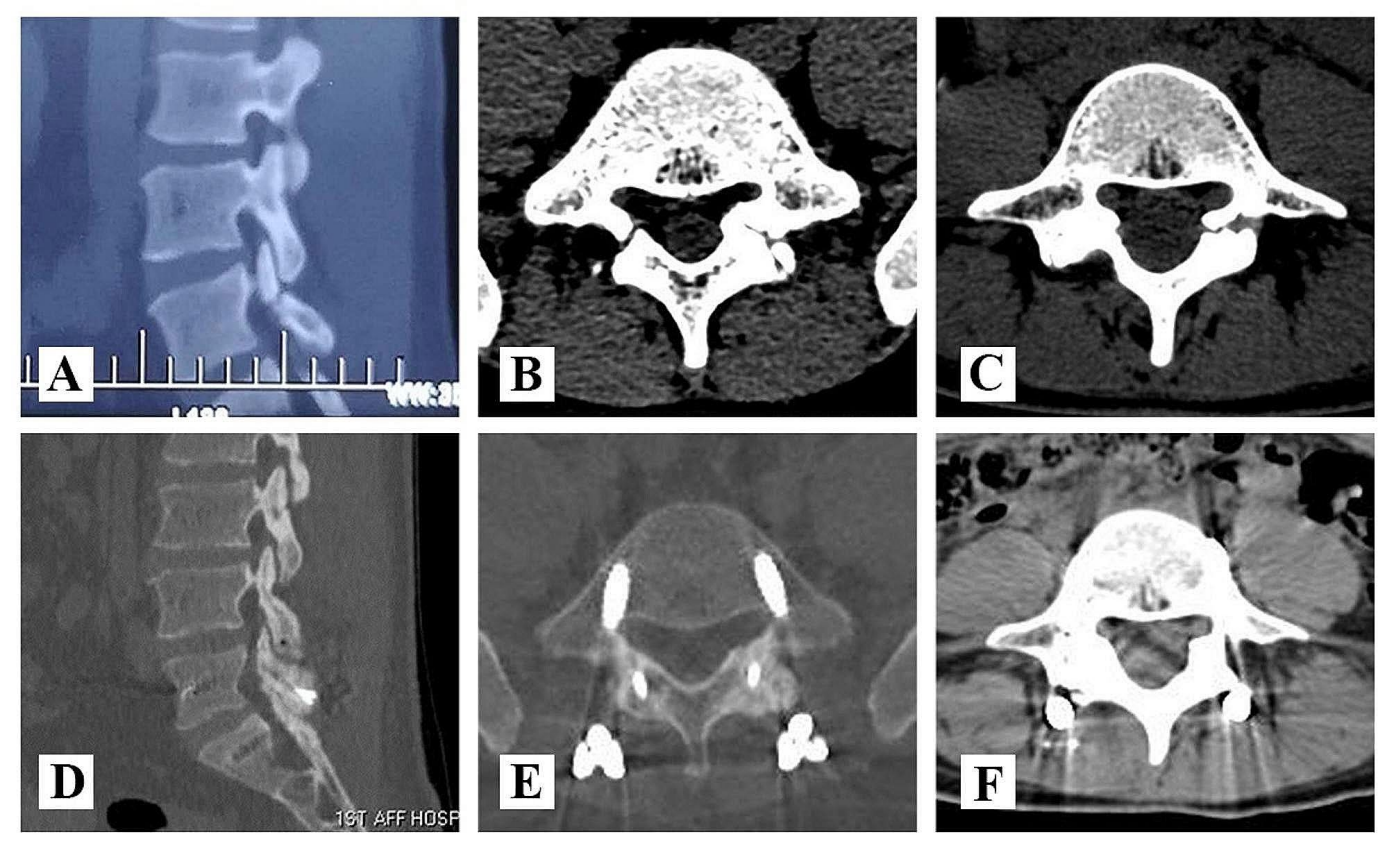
CT indicated satisfactory isthmus fusion. (A, B, C) Preoperative CT imaging data of the patient. (D, E, F) Postoperative CT showed bony fusion
